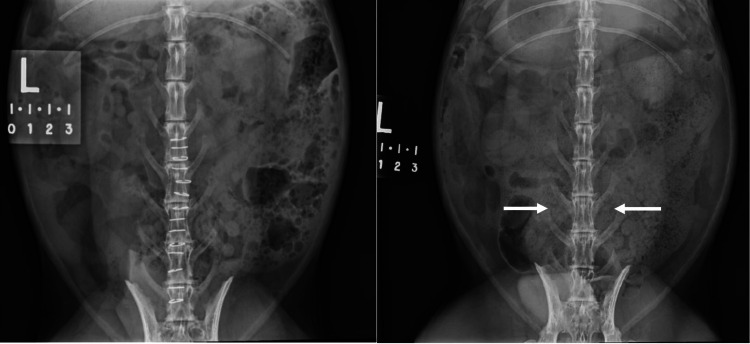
Anteroposterior radiographs obtained immediately postoperatively (left) and at 12 weeks (right). The arrows on the radiograph from 12 weeks’ follow-up demonstrate nonfusion and complete disappearance of the immunologically activated graft.
